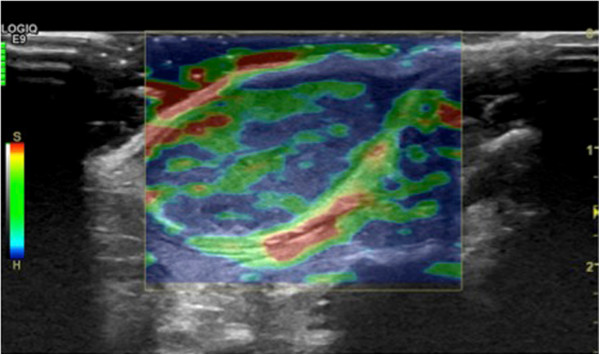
Ultrasound elastography (blue: hard tissue, green: soft tissue): Central tumor necrosis is encoded in green. Due to the immediate vicinity of subcutaneous tissues to the transducer, subcutaneous tumor parts under compression are also coded in green. Further developments investigate elastography without manual compression to minimize these artifacts.
